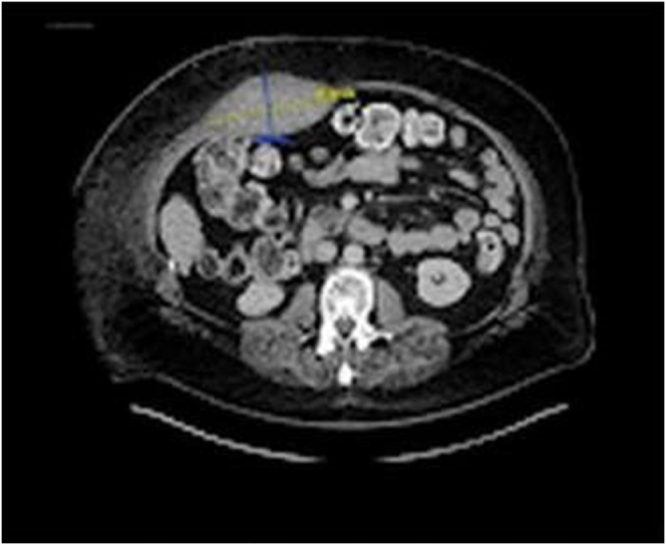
CT scan showing hematoma. CT, computed tomography
